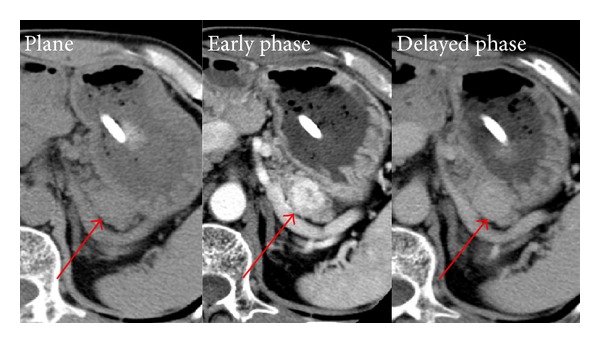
Abdominal computed tomography revealed a hypervascular tumor (arrow) in the middle portion of the pancreas in April 2012.
